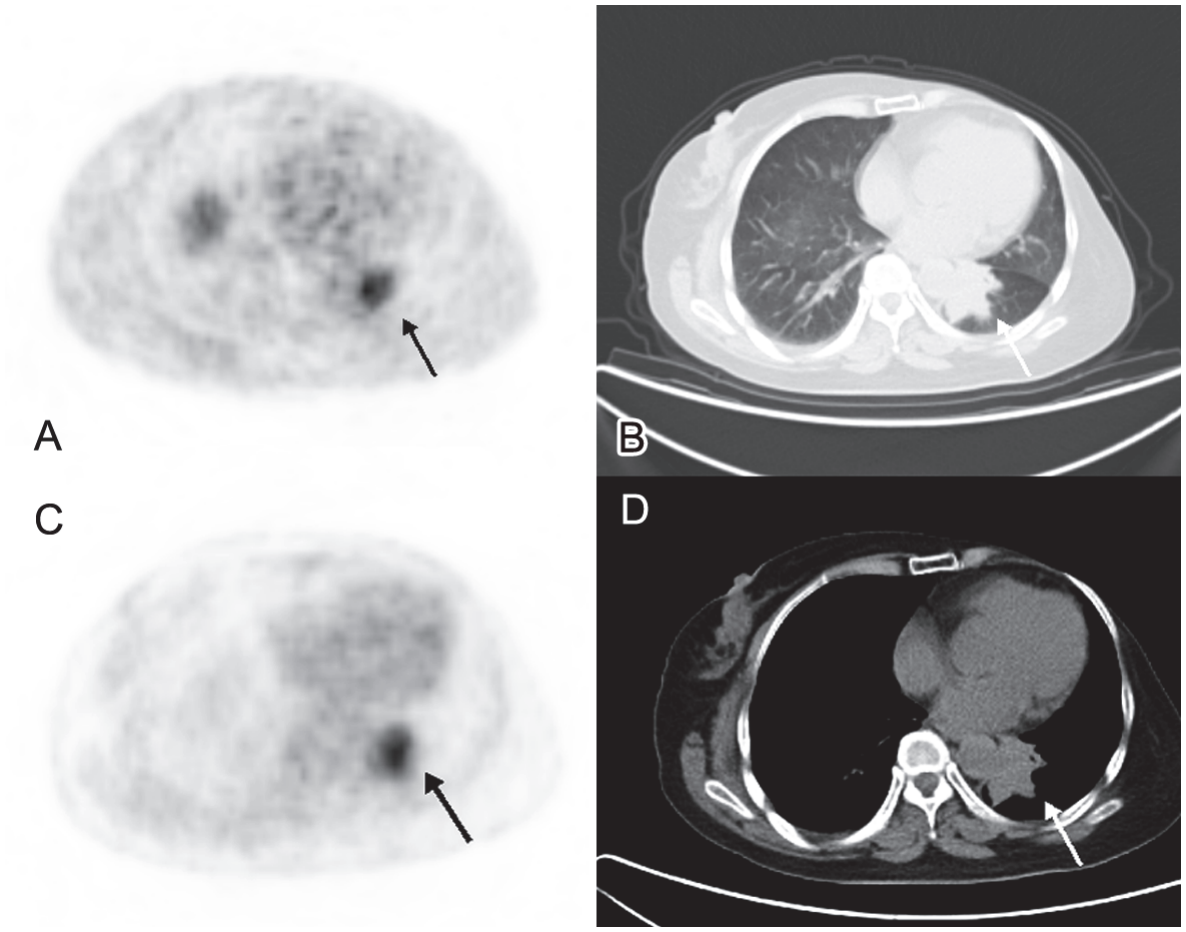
A 58-year-old female breast cancer patient. We detected a mass with irregular margin in left lung (B,D. CT imaging), with a maximum diameter of 4.5cm. It was difficult to differentiate it between metastasis and secondary primary lung cancer in CT imaging. The tumor presented with high uptake of both ^18^F-FES and ^18^F-FDG PET, SUVmax was 6.3 and 5.5 respectively. It suggested that it was a metastasis (A. ^18^F-FES PET/CT, C. ^18^F-FDG PET/CT). After operation, it was confirmed to be an ER positive metastasis from breast cancer.
